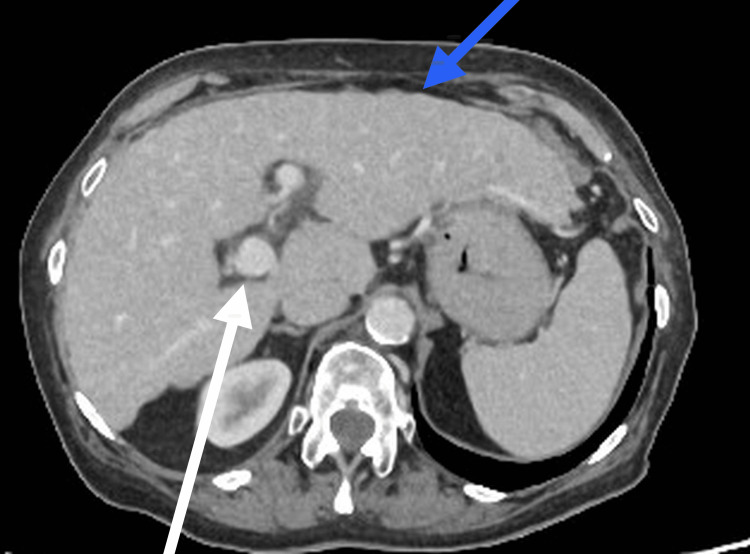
Contrast-enhanced CT of the abdomen showing nodular liver contour (blue arrow) with features of portal hypertension, including recanalisation of the umbilical vein (white arrow) CT: computed tomography
